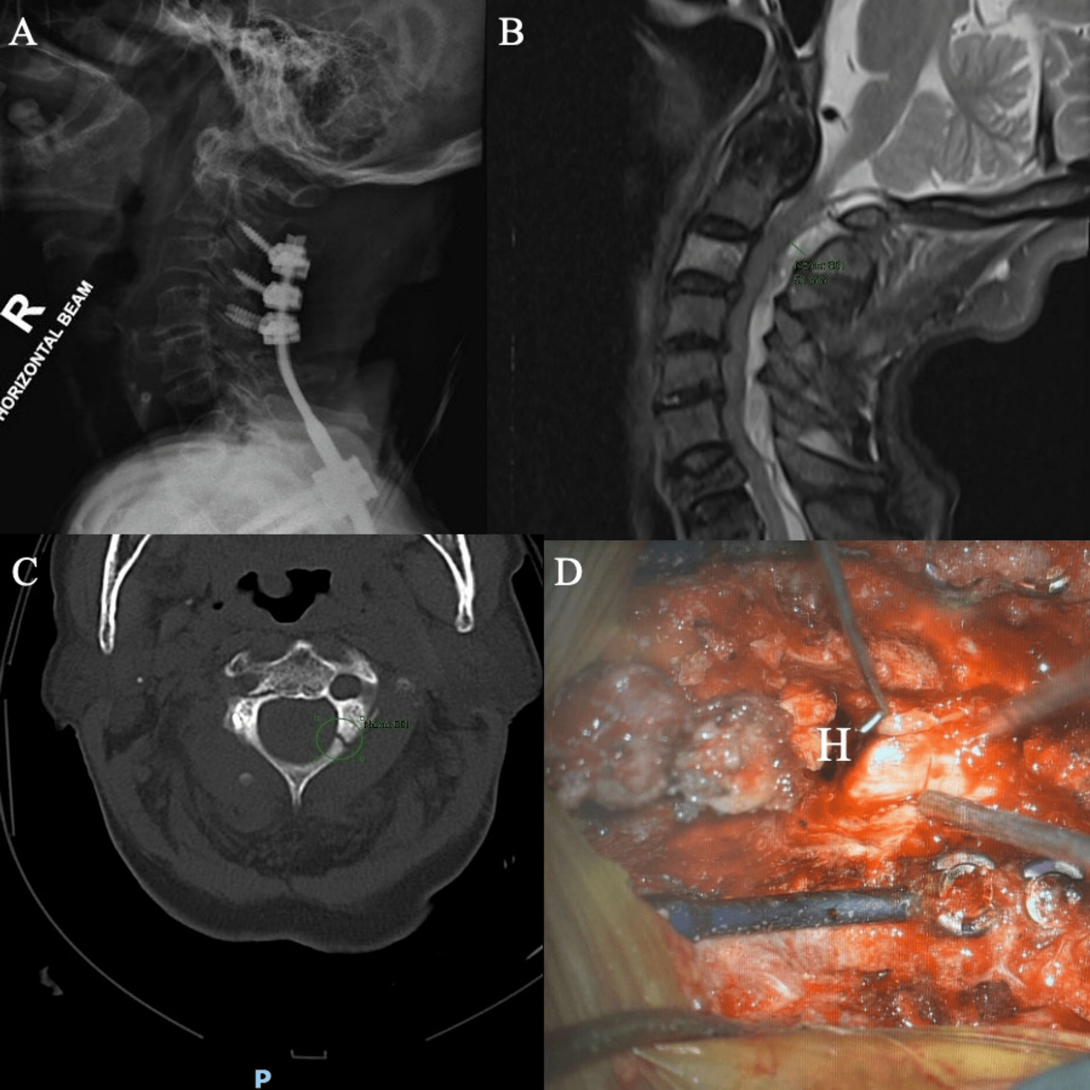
Case 3 (A) Lateral X-ray of the cervical spine showing C2-T4 posterior instrumentation for right C6/7 facet dislocation. (B) CT cervical spine showing minimally displaced C2 (atypical hangman) fracture with loosened left C2 screw. (C) MRI-CS showing an extensive epidural hematoma spanning from foramen magnum to T1 with cord edema at the C4-C6 levels and multi-level stenosis at the C3-C6 levels. (D) Intraoperative photograph with hematoma shown (labeled “H”)
